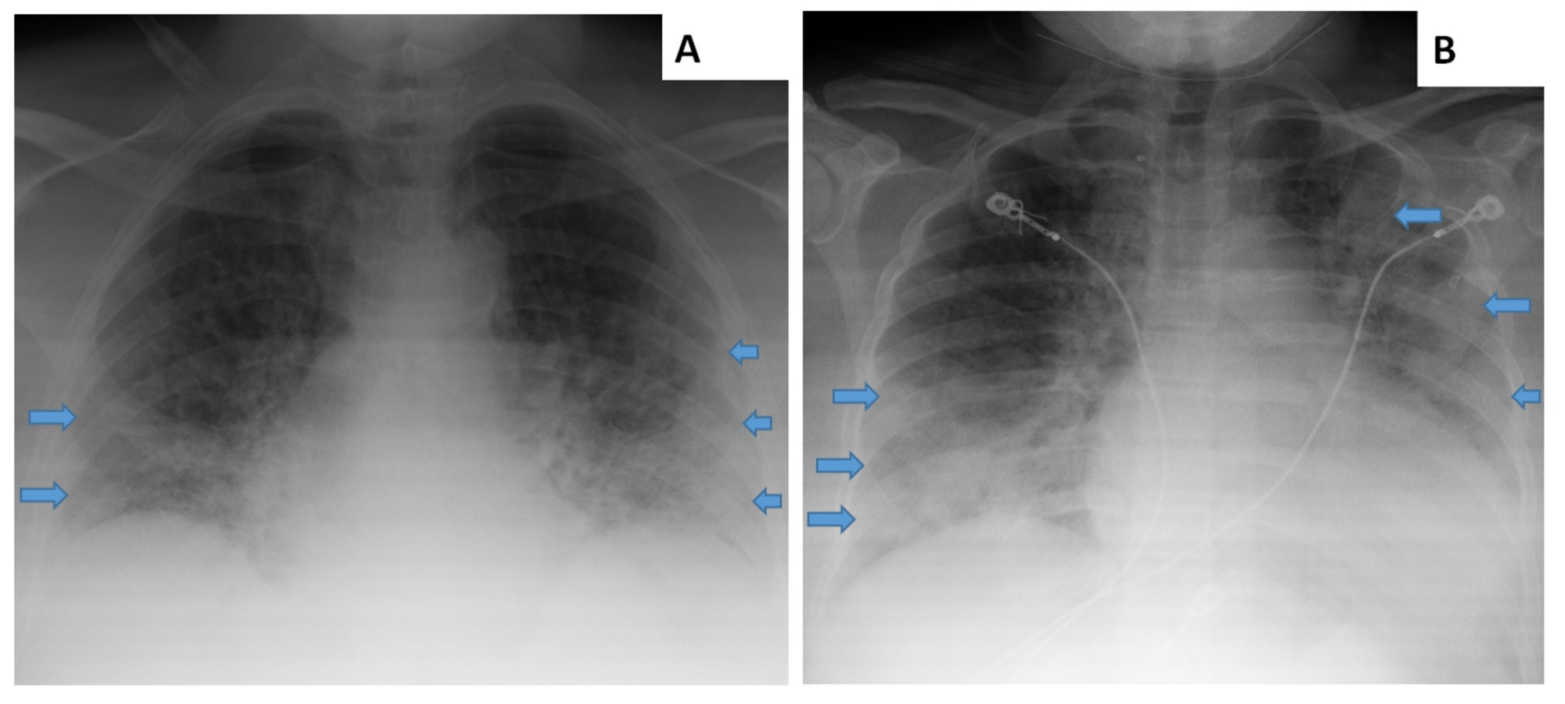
Chest X-rays of Patients A and B. Arrows indicate some portions of affected regions.
